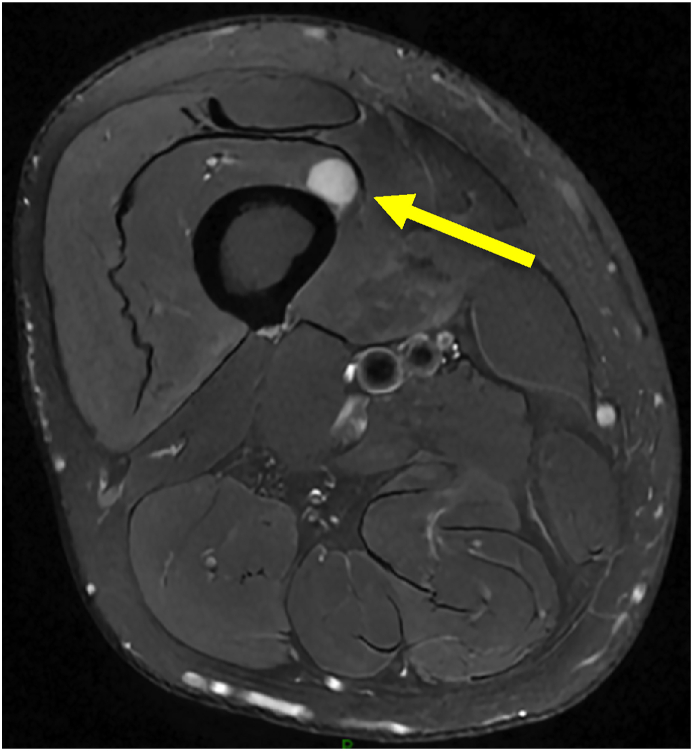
MRI of the lower extremity showing a 1.2 cm schwannoma in the vastus intermedius.
